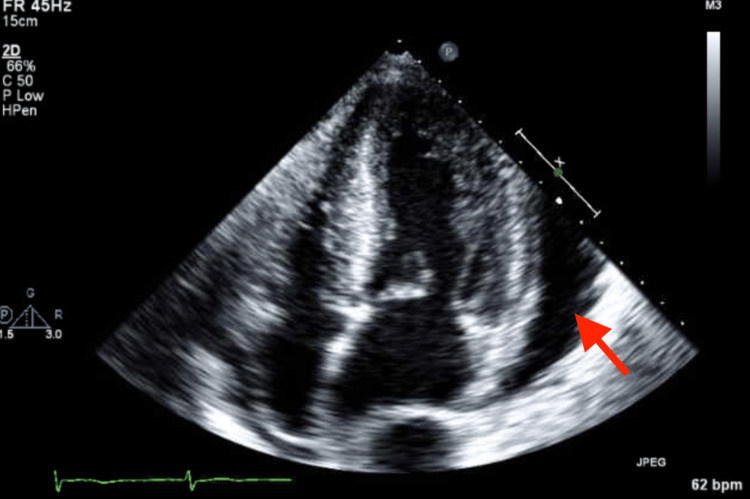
Transthoracic echocardiogram, apical four chambers view, at the time of admission demonstrating large pericardial effusion at the LV lateral wall and RV free wall and increased LV wall thickness The red arrow points to pericardial effusion.
